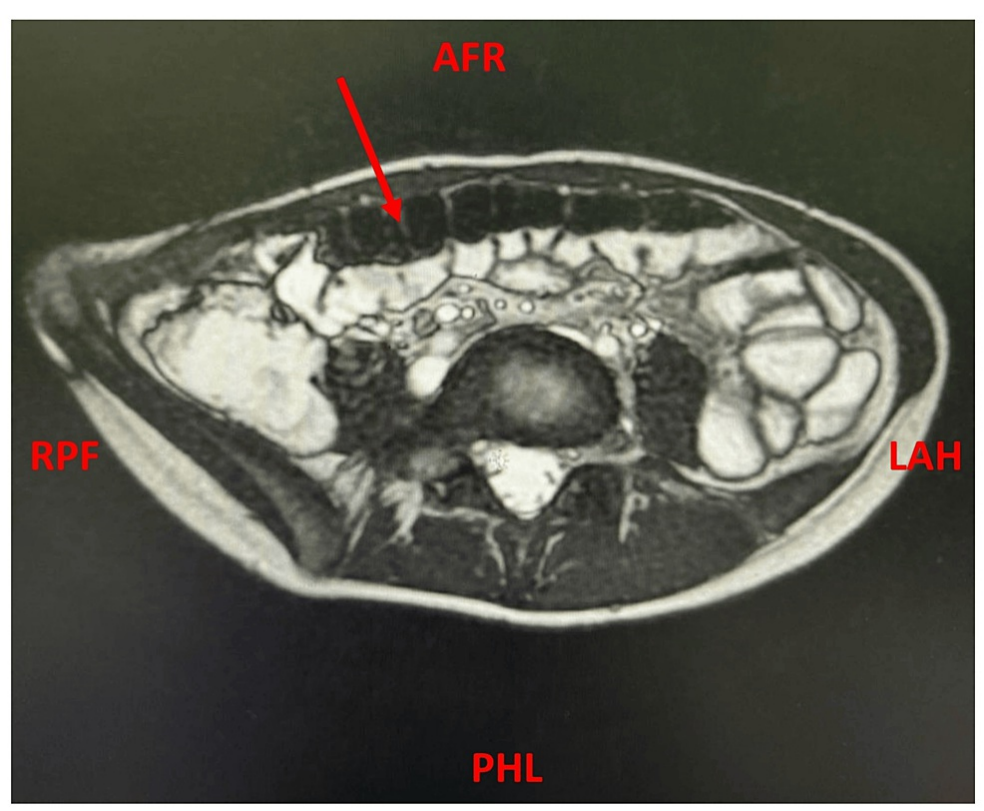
The magnetic resonance imaging (MRI) enterography of the studied patient The red arrow indicates abnormal bowel motion. AFR - anterior-foot-right; PHL - posterior-head-left; RPF - right-posterior-foot; LAH - left-anterior-head
